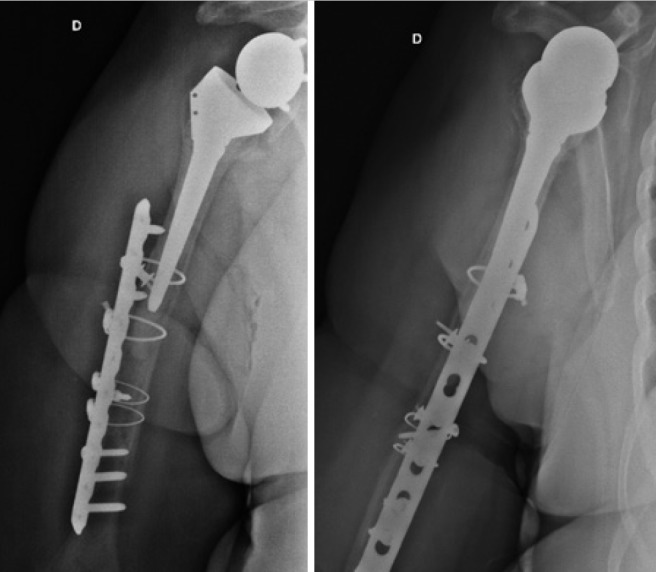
Front and profile radiography, showing fracture healing
